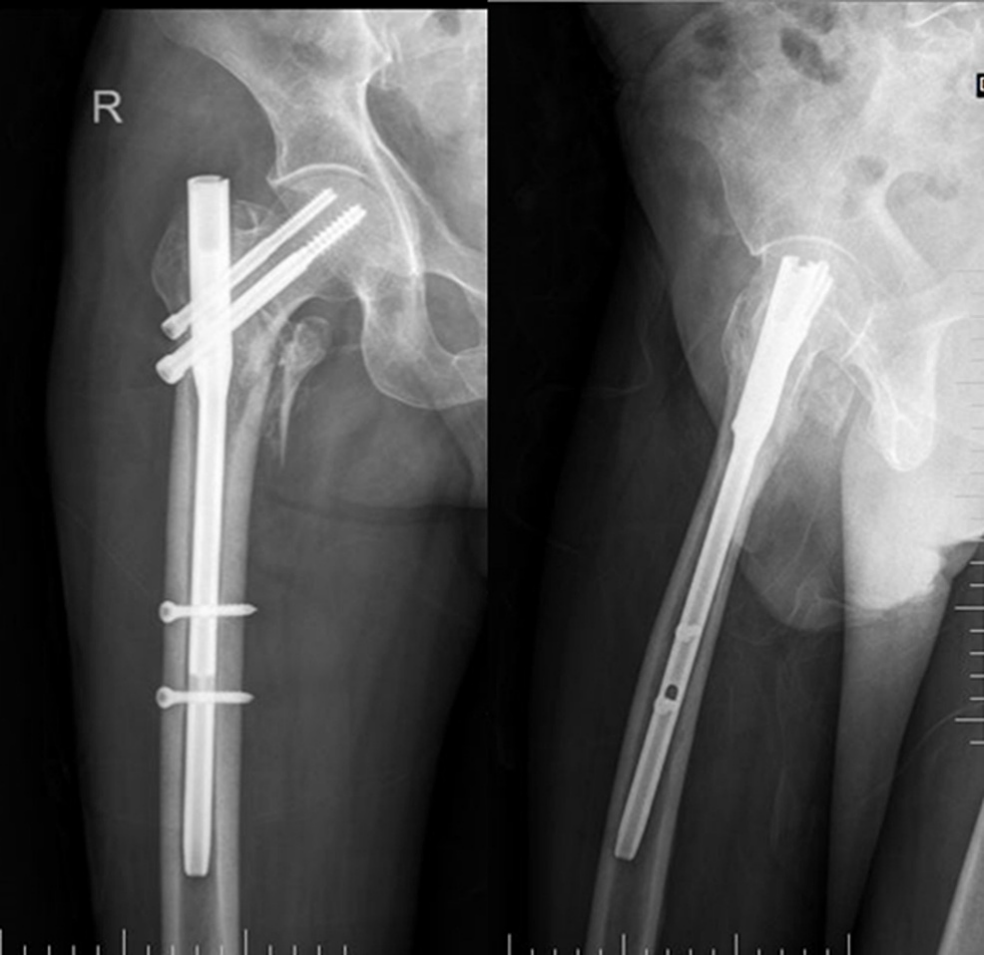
Six weeks follow-up - right hip AP (L) and lateral (R) radiographs AP: anteroposterior
